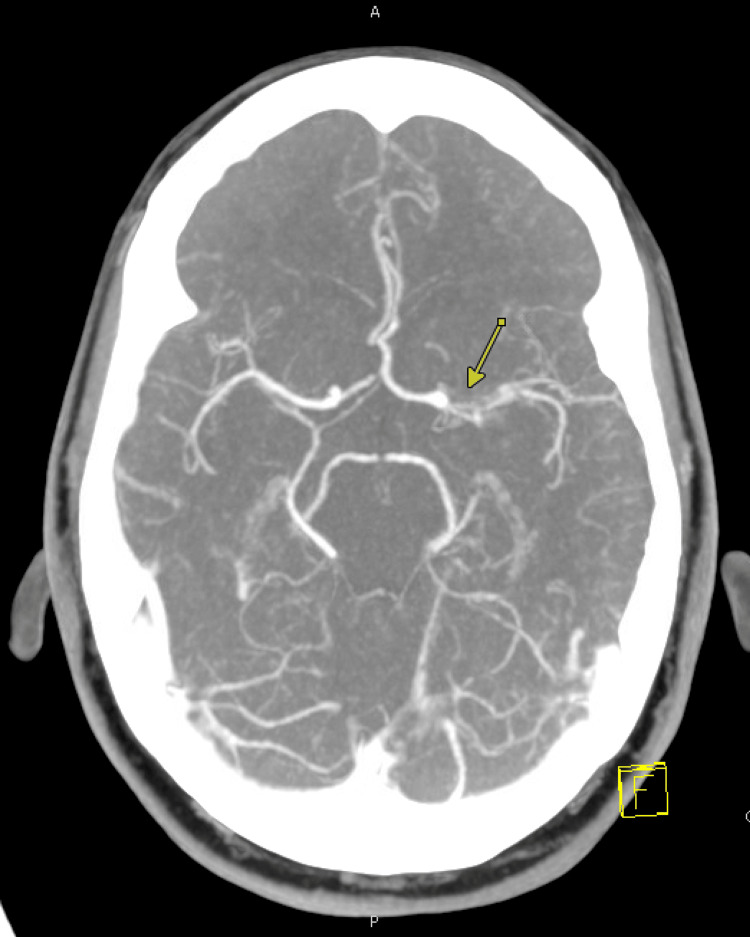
CTA Head With a Chronically Occluded Left MCA as Demonstrated by the Yellow Arrow CTA: Computed Tomography Angiography; MAC: Middle Cerebral Artery
